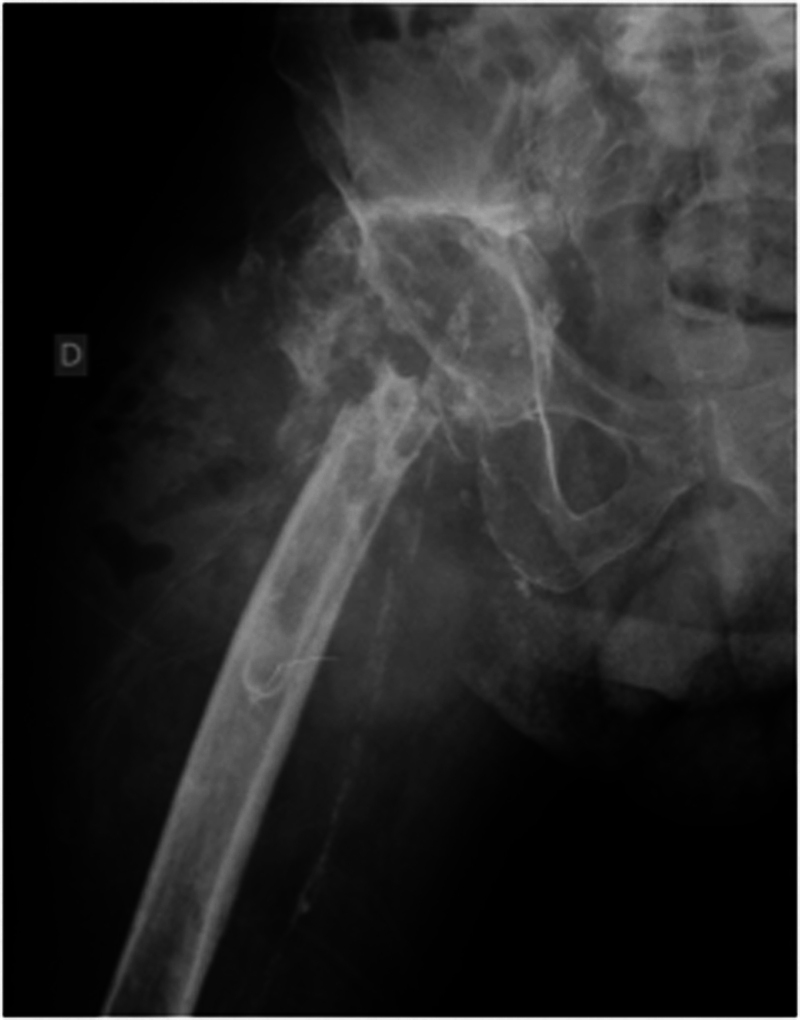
Right hip radiograph performed in July 2016 after revision arthroplasty procedure through posterolateral access with the removal of prosthetic components. Presence of a large tumoral mass in soft tissues in association with extensive destruction of the proximal third of the femur.
